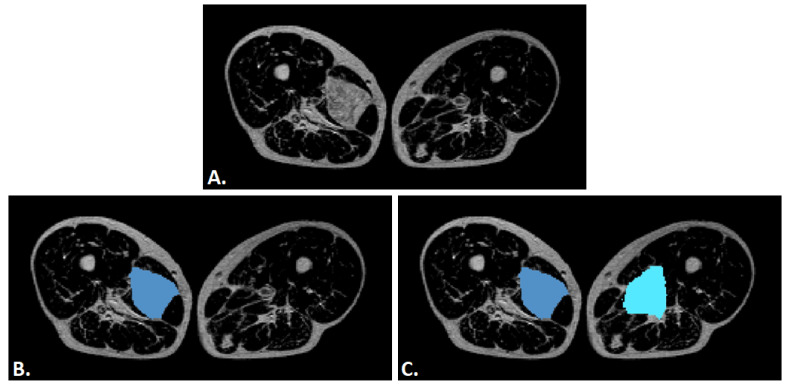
Example of tumor and healthy tissue segmentation in axial T1-weighted MRI of the thighs. (A) shows the original MRI with a unilateral soft tissue sarcoma. (B) displays the manual segmentation of the tumor (blue), while (C) shows the corresponding healthy tissue regions (light blue) from the contralateral limb, used as negative samples.
